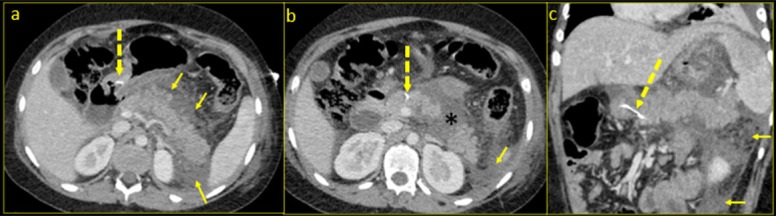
Axial CECT at the level of body of pancreas (a), at the level of head and body caudal to section 1a (b) and coronal-curved MPR images (c). The pancreas is bulky and oedematous with fat stranding and fluid in the lesser sac, left pararenal and lateral conal spaces (small yellow arrows). A focal area of necrosis in the form of parenchymal non-enhancement (black Asterix in b) is seen in the body of pancreas. The dashed yellow arrow in Figure 1a, b and c points to a curvilinear high density foreign body, whose proximal end is in the antropyloric canal of stomach (a) and distal end in embedded in the head of pancreas (b). CECT, contrast-enhanced CT; MPR, multiplanar reconstruction.
